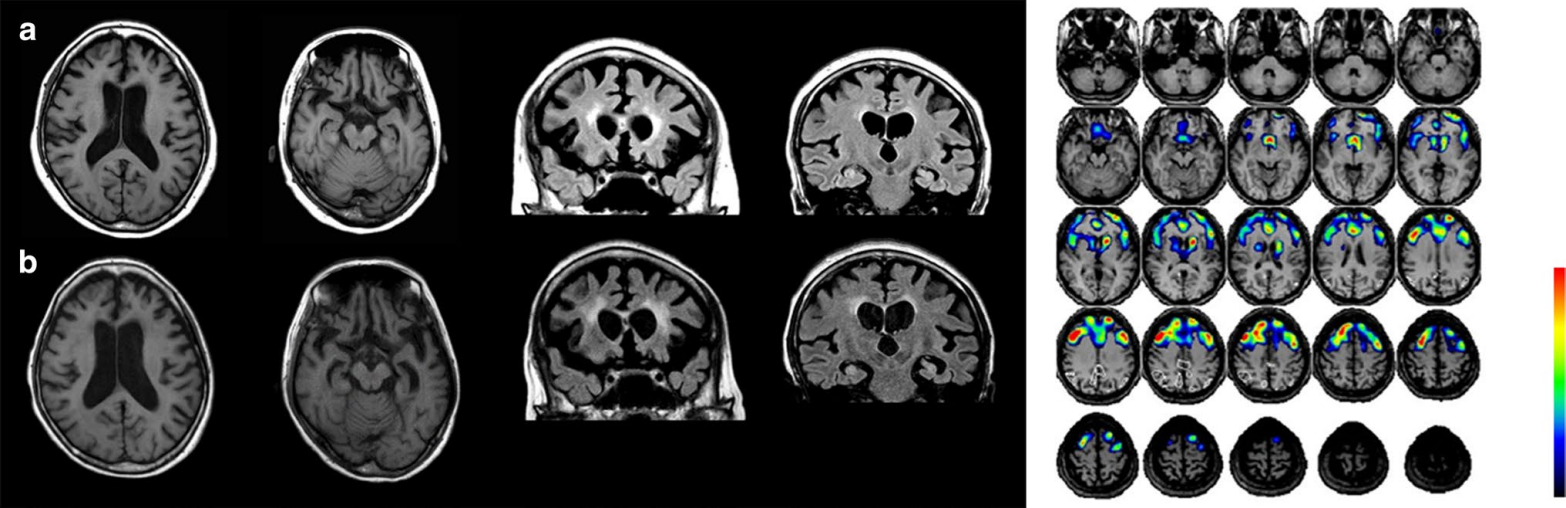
Imaging of an FTLD-TDP patient showing the clinical presentation of naPPA. Left photo shows T1-weighted MRI at onset (a) and 2 years after onset (b). Frontal and temporal atrophy on axial slices (two photos on the left) is prominent. Coronal slices (two photos on the right) indicate atrophy in the dorsolateral prefrontal cortex and medial and inferior temporal cortex with enlargement of the temporal horn. Single-photon-emission computed tomography using technetium-99 m ethyl cysteinate dimer (ECD-SPECT) revealed a reduction in the bifrontal regions (Right photo)
